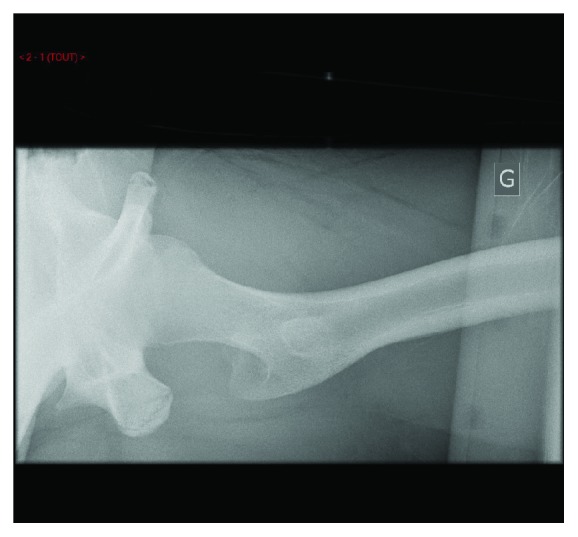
Emergency left hip X-ray axial view.
